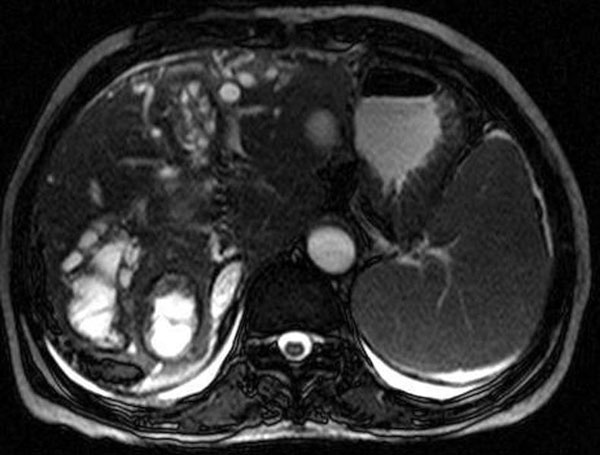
Axial T2-weighted MRI showing hepatic hydatid cysts and changes secondary to operation.
